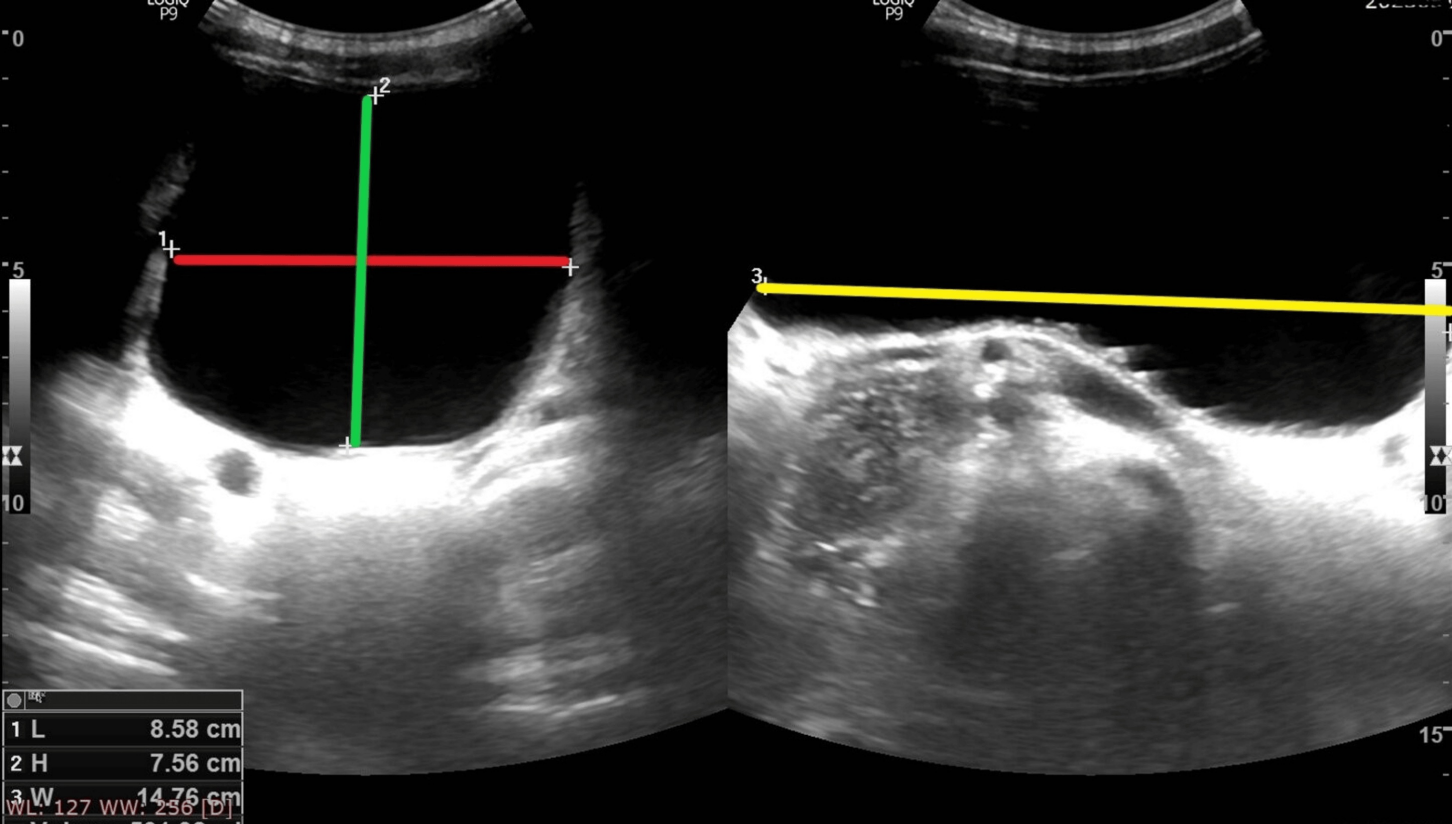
Ultrasonography of the pelvis showing significant post-void residual urine volume. The red, green, and yellow lines respectively indicate the length, height, and width of the urinary bladder.
